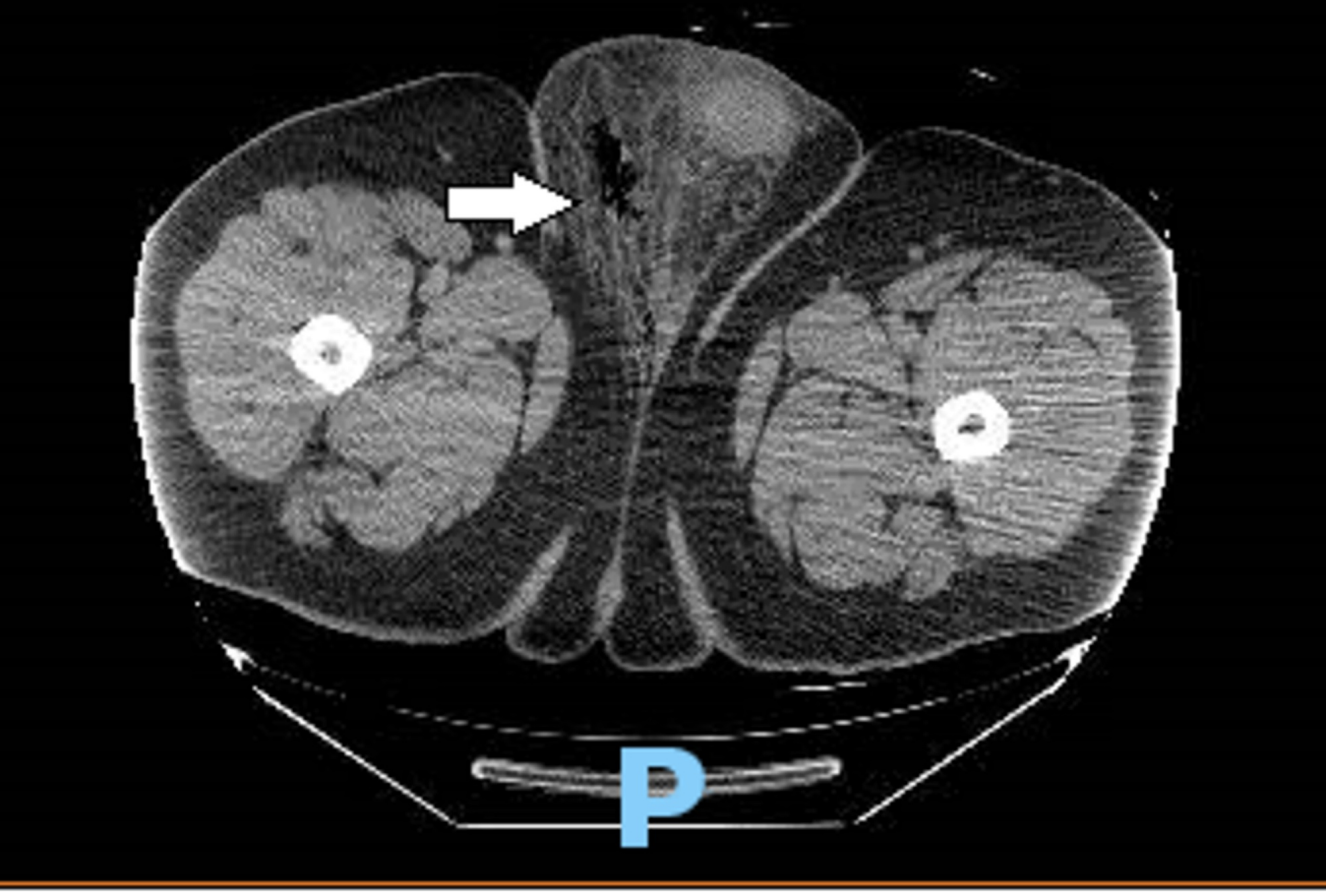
Computed tomography of abdomen and pelvis showing inflammatory changes and gas noted in the right inguinal region with extension into the right side of the scrotum and scrotal edema.
